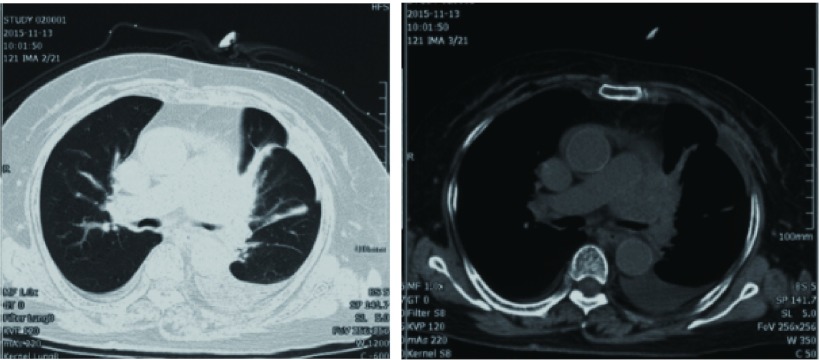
与4月余前CT片比较，左主支气管前软组织影较前增大，左上叶支气管管腔已闭塞，伴有阻塞性肺炎，左侧胸腔积液较前增多，并出现少量心包积液及右侧胸腔积液（2015-11-13）。 Compared with the CT of four months ago, soft tissue shadowbefore left main bronchus increase. Left upper lobe bronchial lumen is occlusive, accompanied with obstructive pneumonia. Left pleural effusion increase, and a small amount of pericardial effusion, and right pleural effusion (2015-11-13).
